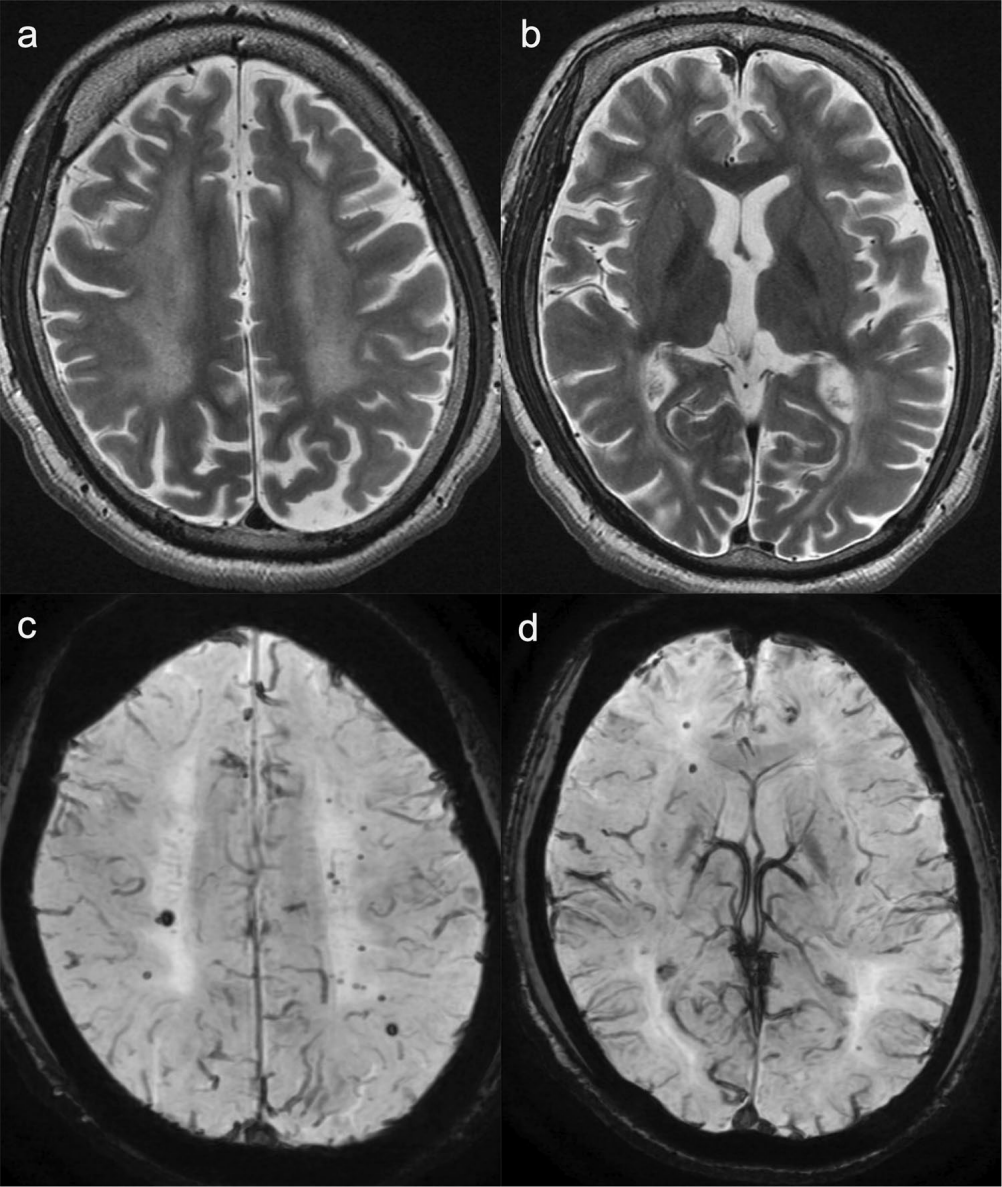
5,10-methylene-tetrahydrofolate reductase deficiency (MTHFRD). An 18-year-old, male patient with epilepsy, intellectual disability, and autistic tendency. The patient had lower limb motor weakness and urinary incontinence for four years. a, b T2-weighted images demonstrated mild brain atrophy and white matter hyperintensities with posterior predominance. c, d SWAN demonstrated multiple, cerebral microbleeds in the white matter (Source: reference 55)
